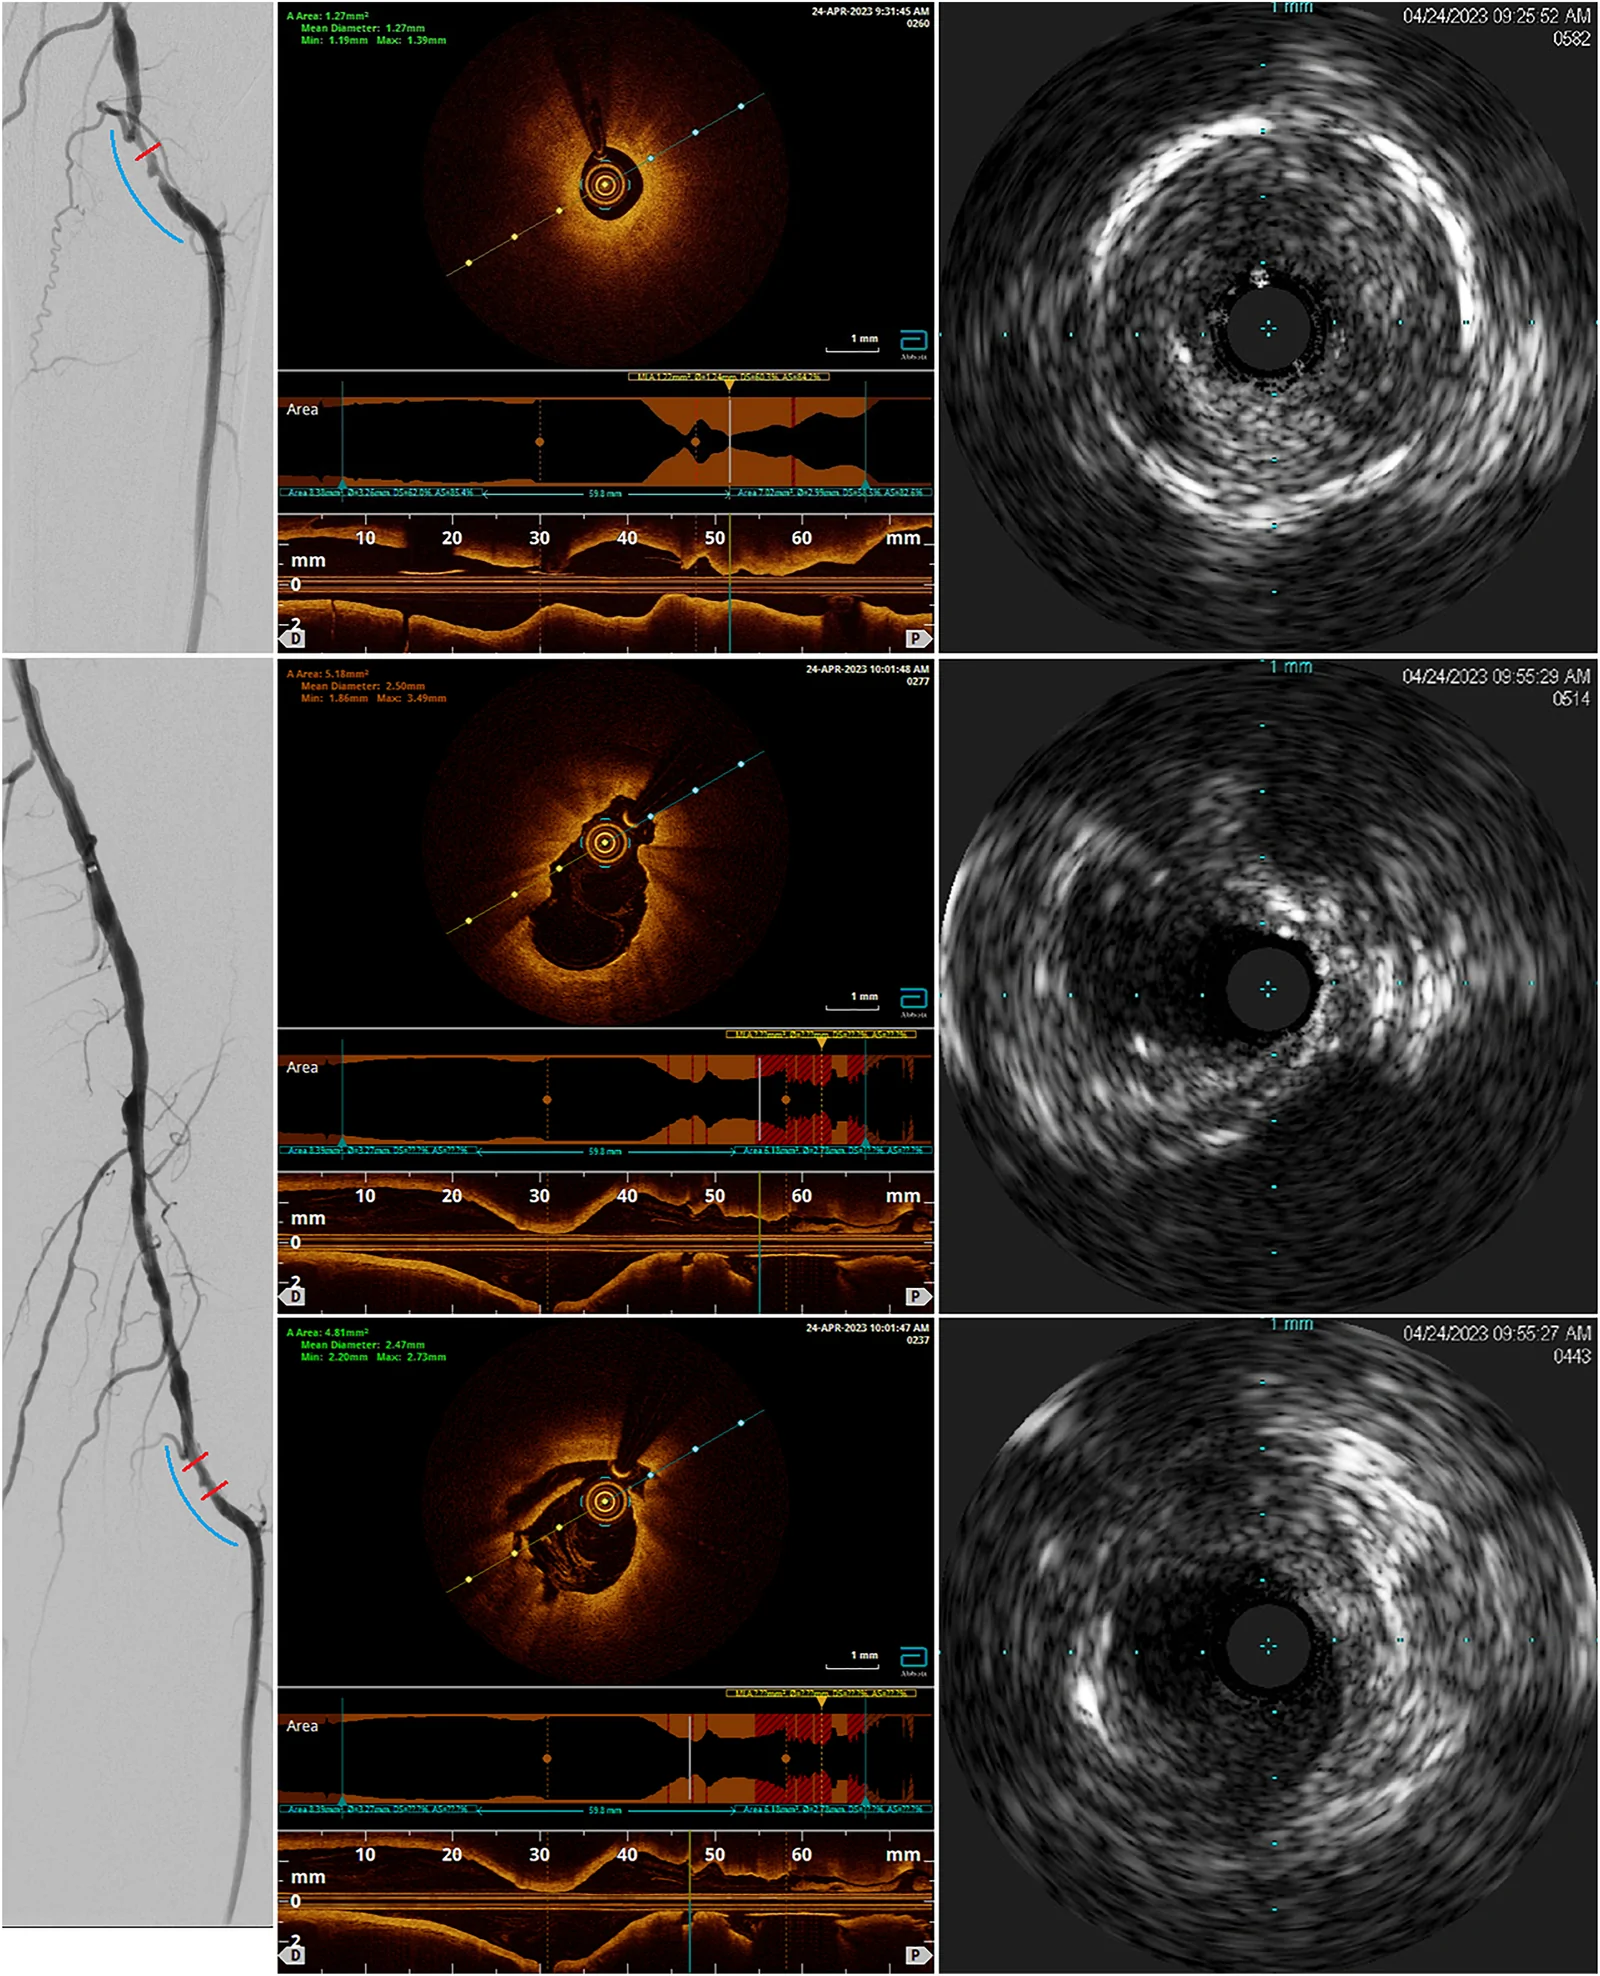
A patient with severe proximal anterior tibialdisease and occlusion of the tibioperoneal trunk, posterior tibial, and peroneal artery. Preangiograms and postangiograms with corresponding optical coherence tomography (OCT) and intravascular ultrasound (IVUS) frames are shown. The region of interest is marked by a blue arc; red lines indicate OCT and IVUS cross-sections. In the preintervention image (top panel), the lesion appears highly fibrotic on OCT and heterogeneous on IVUS. IVUS shows adventitial calcium not clearly visible on OCT because of attenuation from fibrolipid-rich plaque. Postintervention OCT shows reasonable lumen gain in proximal anterior tibial artery (mid panel), but a dissection flap is seen distally (bottom panel). IVUS visualization of the lumen and flap is less distinct.
